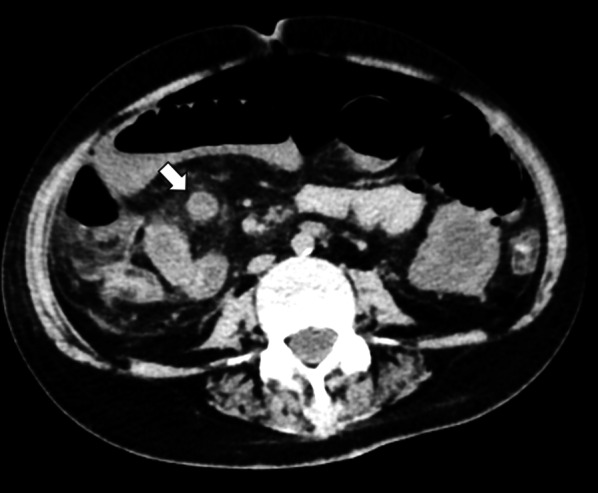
Computed tomography coronal view image obtained free air and a right quadrant heterogeneous mass within the loop of ileum (close arrows)
